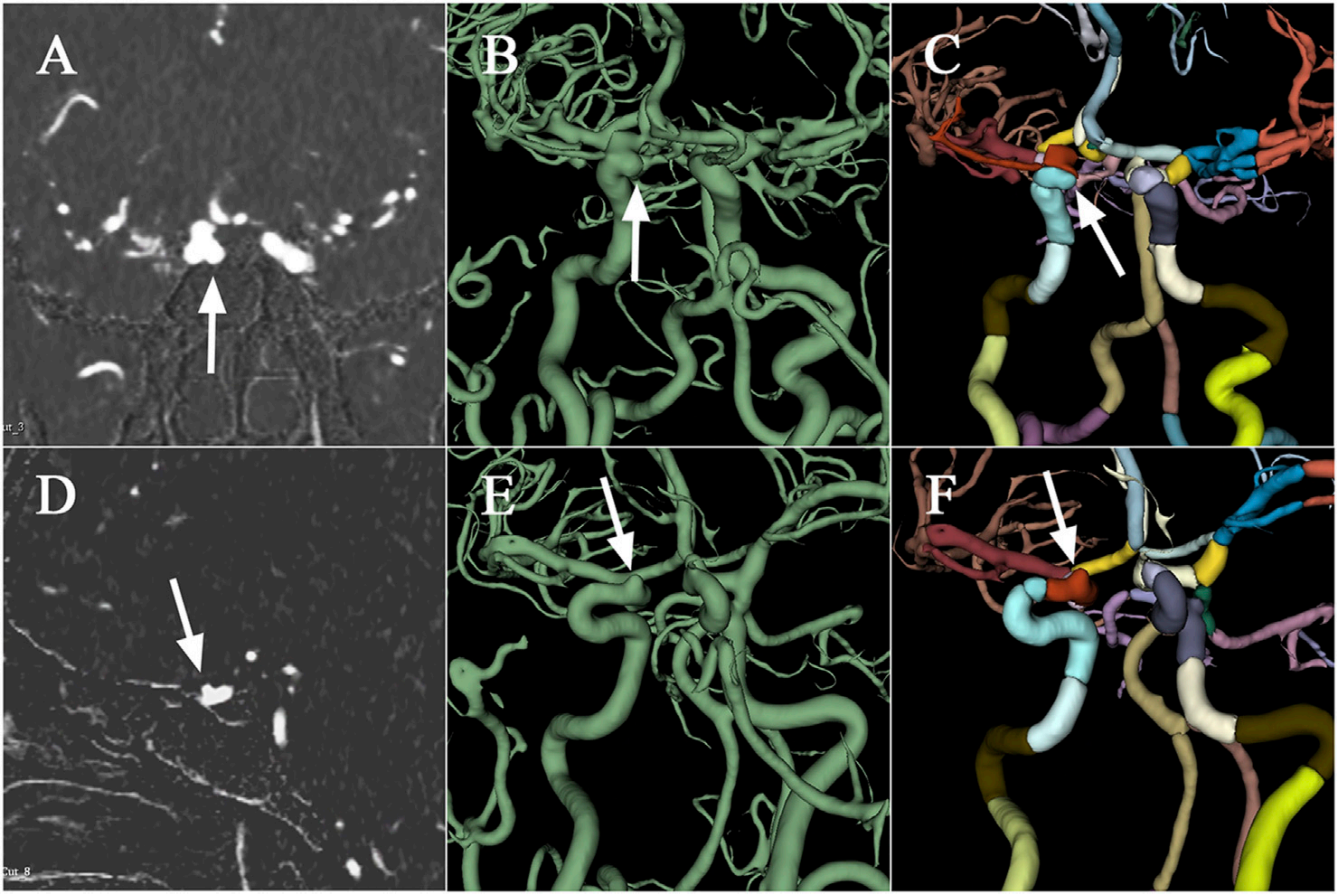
Examples of aneurysms in the validation dataset. The first raw presents CTA scan (A), binary mask (B), and the labelled arteries (C) with IA located on R-C5 of ICA, which are displayed from left to right column. Similarly, the second raw depicts CTA scan (D), binary mask (E), and the labelled arteries (F) with IA located on R-C6. CTA, computed tomography angiography; IA, intracranial aneurysm; ICA, internal carotid artery; R, right.
